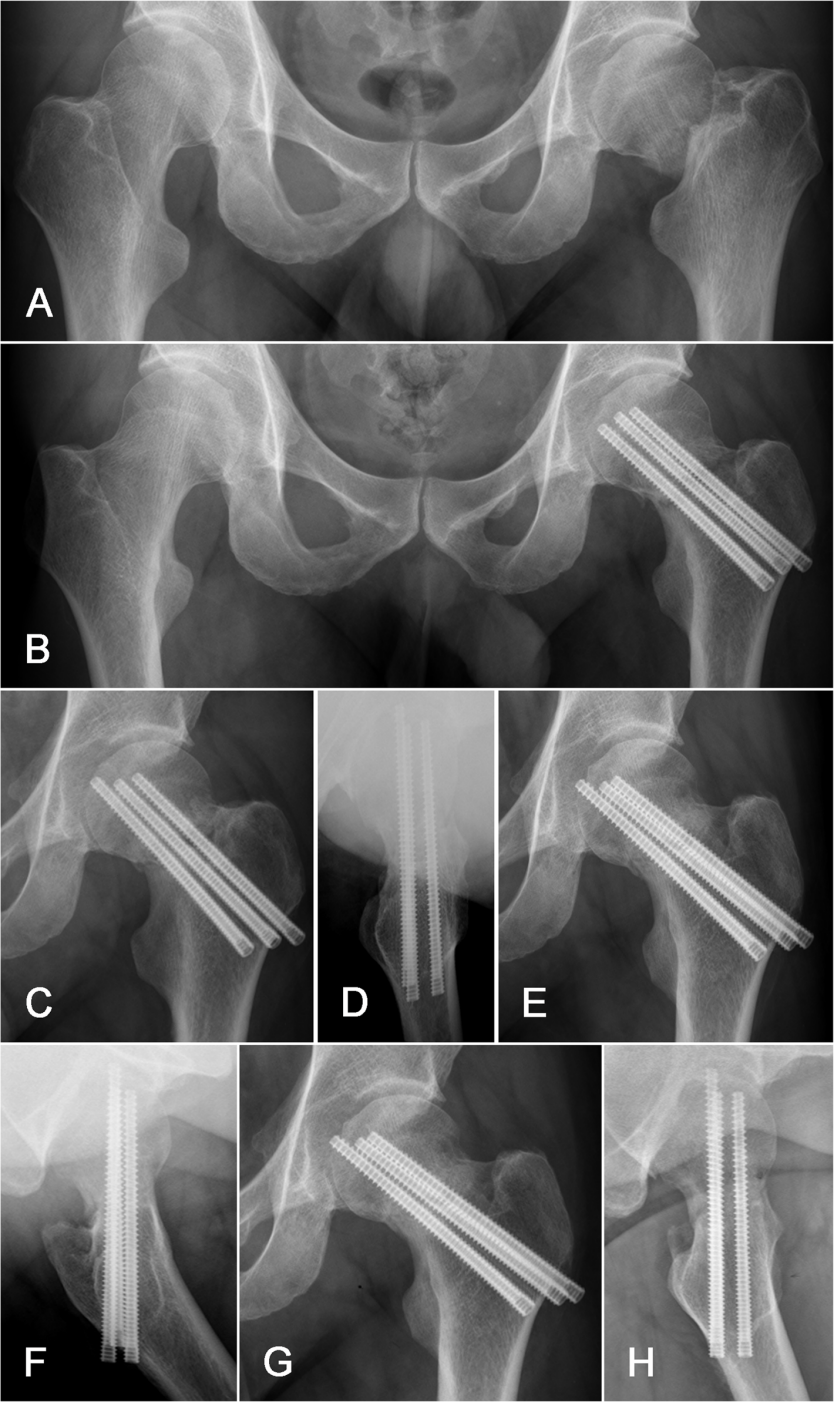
FTHCS fixation for FNF in a 43-year-old male complicated by medial screw migration. a AP radiograph of the pelvis including both hip joints revealed a Garden type IV, Pauwels type II left femoral neck fracture with VN angle < 15°. b Immediate postoperative radiographs showed acceptable reduction and three FTHCS. AP and lateral radiographs 3 months postoperatively (c, d) demonstrated migration of the distal screw beneath the femoral head subchondral bone in the direction of the acetabulum with slight fracture displacement. Radiographs 4 months postoperatively (e, f) exhibited further displacement of the distal screw tip, penetrating the subchondral bone. Six-month postoperative radiographs (g, h) revealed fracture union with no further shift of the displaced screw and the patient was without functional limitations
